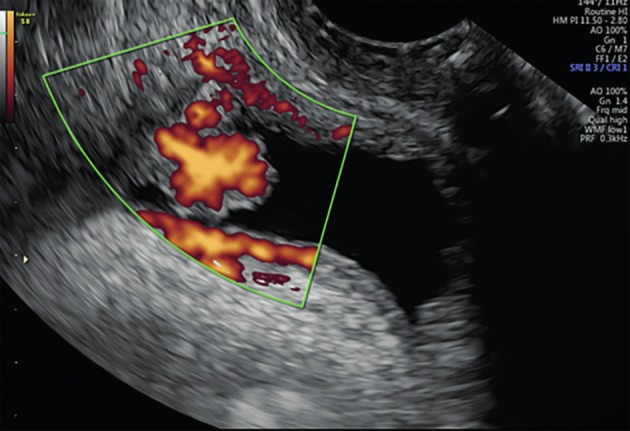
Transvaginal sonography displaying a highly vascularized malignant lesion in the bladderwall.
